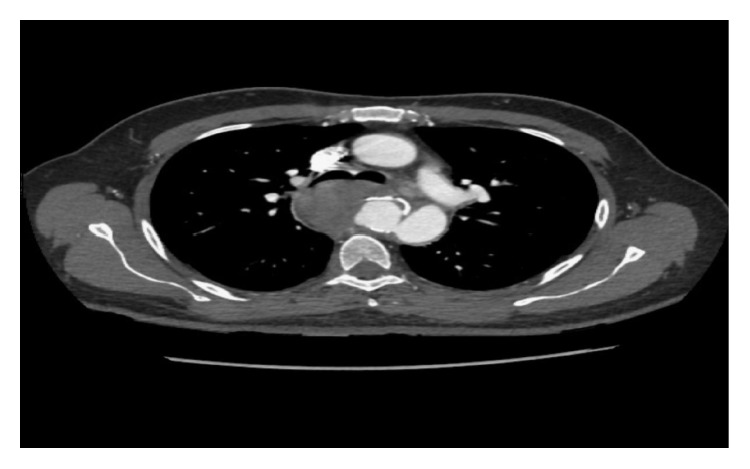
CT chest shows large saccular aneurysm in the proximal part of the descending aorta.
